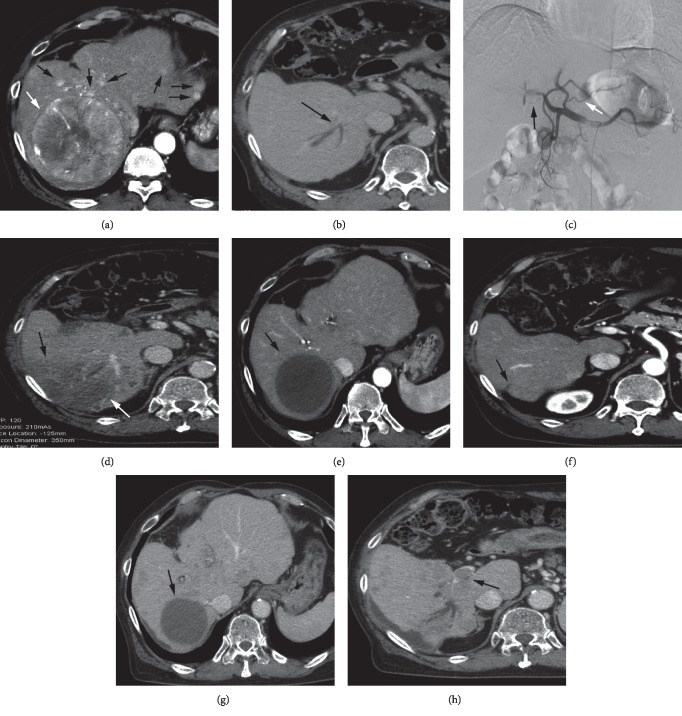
Subsegmental level liver infarction after RAIB-TACE. On arterial phase CT, a large nodule (white arrow, A) and multiple intrahepatic metastases (black arrows, A) were seen. On portal phase CT, dilatation of biliary tract in subsegment 7 (black arrow, B) was seen due to compression of proximal Glisson sheath by the large nodule. RAIB-TACE for the bi-lobe of the liver was done. Post-TACE DSA depicted patent cystic (black arrow, C) and right gastric arteries (white arrow, C) while occlusion of all hepatic arterial branches was achieved. Grade 4 increase of serum ALT level was seen on the next day after RAIB-TACE, and CT obtained 3 days after RAIB-TACE showed low density area (black arrows, D) that was compatible with liver infarction. On CT 3 months after RAIB-TACE, CR of the large nodule (black arrow, E) and disappearance of intrahepatic metastases were also shown. Dimpling of the liver surface (black arrow, F) was seen in the region of liver infarction. On CT 21 months after RAIB-TACE, CR of the large nodule was maintained (black arrow, G), but portal venous tumor thrombosis (black arrow, H) and rib metastases (not shown) were also seen.
